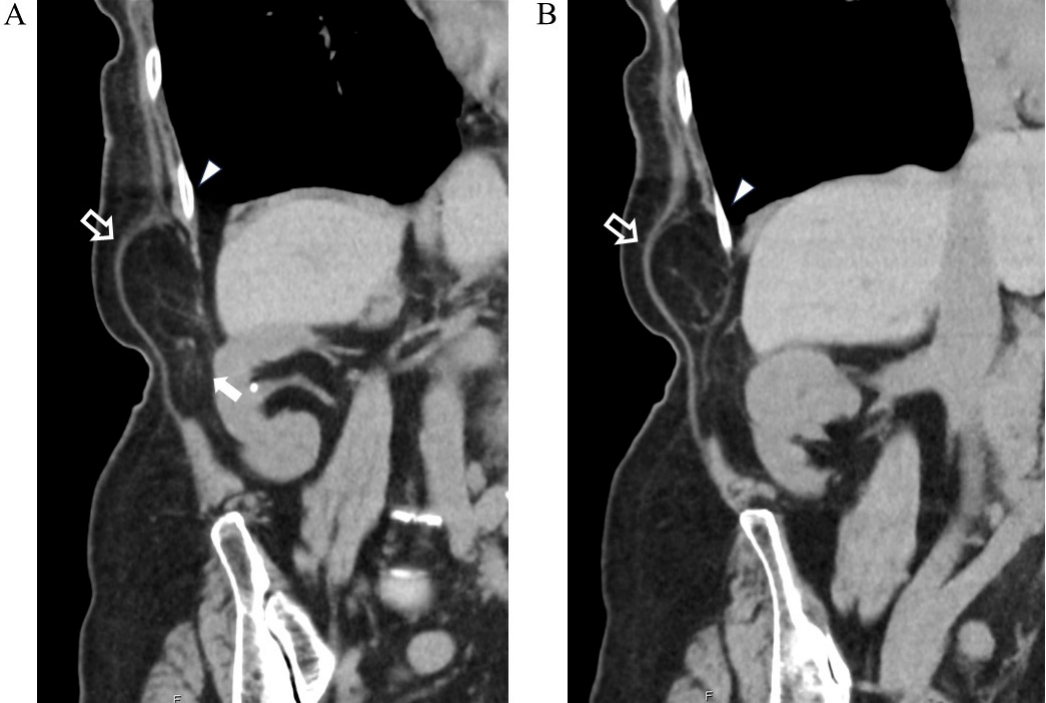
Coronal CT images of the same hernia. (A) The hernial orifice (solid white arrow) and protruding retroperitoneal fat (hollow white arrow) are shown. The fat extends continuously from the retroperitoneal space toward the subcutaneous layer. The 12th rib is also indicated. (B) A more posterior slice than (A), showing the retroperitoneal fat extending upward from the hernial orifice. CT: computed tomography.
